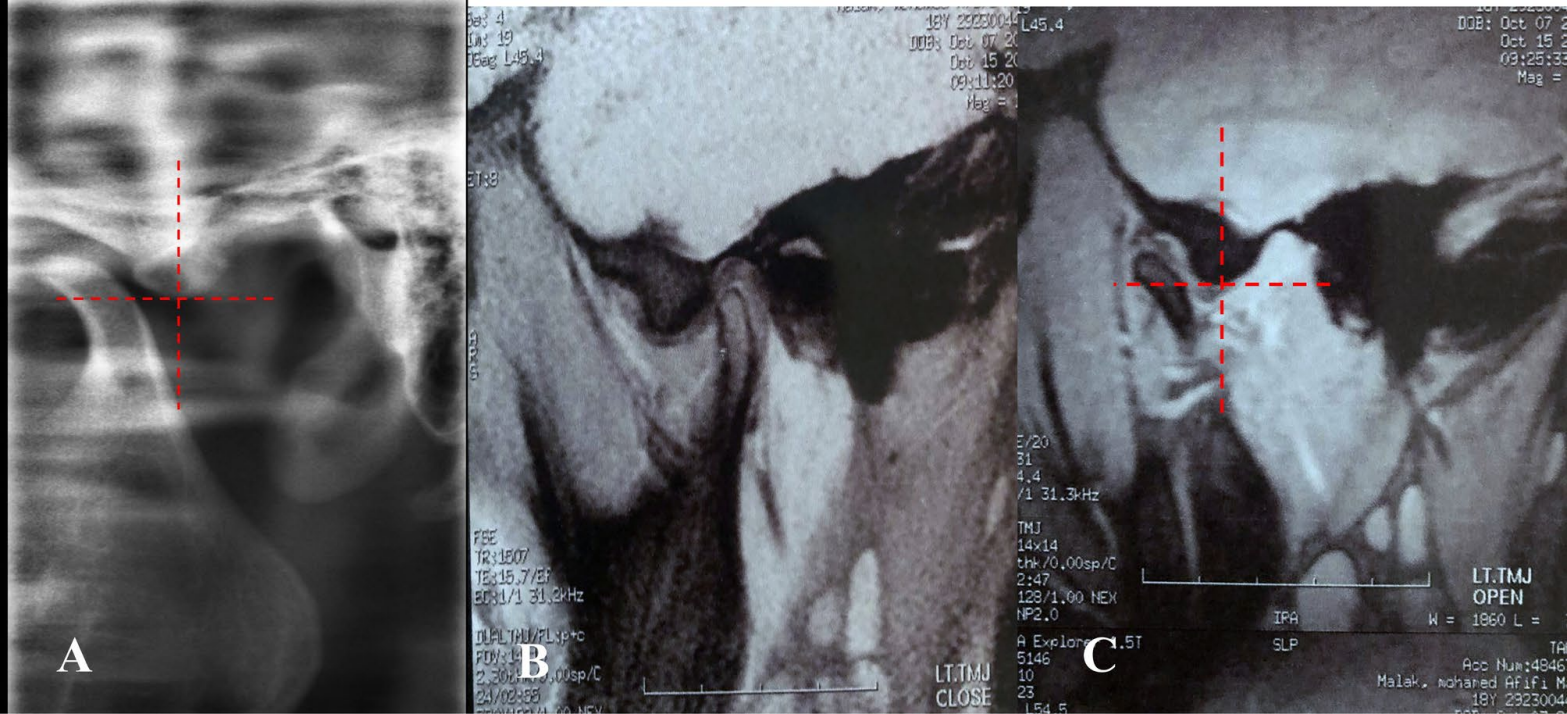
Lateral TMJ view represent Stage III-A (open lock) in open (A) mouth, showing the condyle located anterior to the eminence but at a superior level to the highest point of the eminence. Oblique sagittal T1-weighted images (done after reduction of the open lock) represent Stage III-A, showing a normal condyle-disk-fossa relationship in closed (B) mouth position and in open (C) mouth position. The disk-condyle complex is located in front of the tip of the eminence or high up in front of the base of the eminence.
